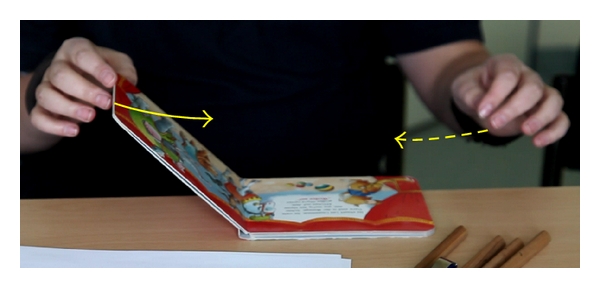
Kallmann's Syndrome. Synkinesia in a patient with KAL1 mutation (c.120_121insC; 122_127del, p.Ala41Glyfs43). When closing a book with one hand (arrow), typical involuntary mirror movement (synkinesia) of the other hand is observed (dotted arrow). This 15-year-old boy presented because of absent puberty. There was orchidopexy at the age of 2 years, disturbed spatial orientation, retarded fine-motor developmental milestones, and anosmia. Tanner P1, G1. LH <0.1 U/L, FSH 0.4 U/L, testosterone 0.2 ng/mL. During GnRH stimulation test, LH 0.5 U/L, FSH 2.1 U/L after 60 minutes. Cranial MRI revealed agenesis of olfactory bulbs and a normal-sized pituitary gland.
